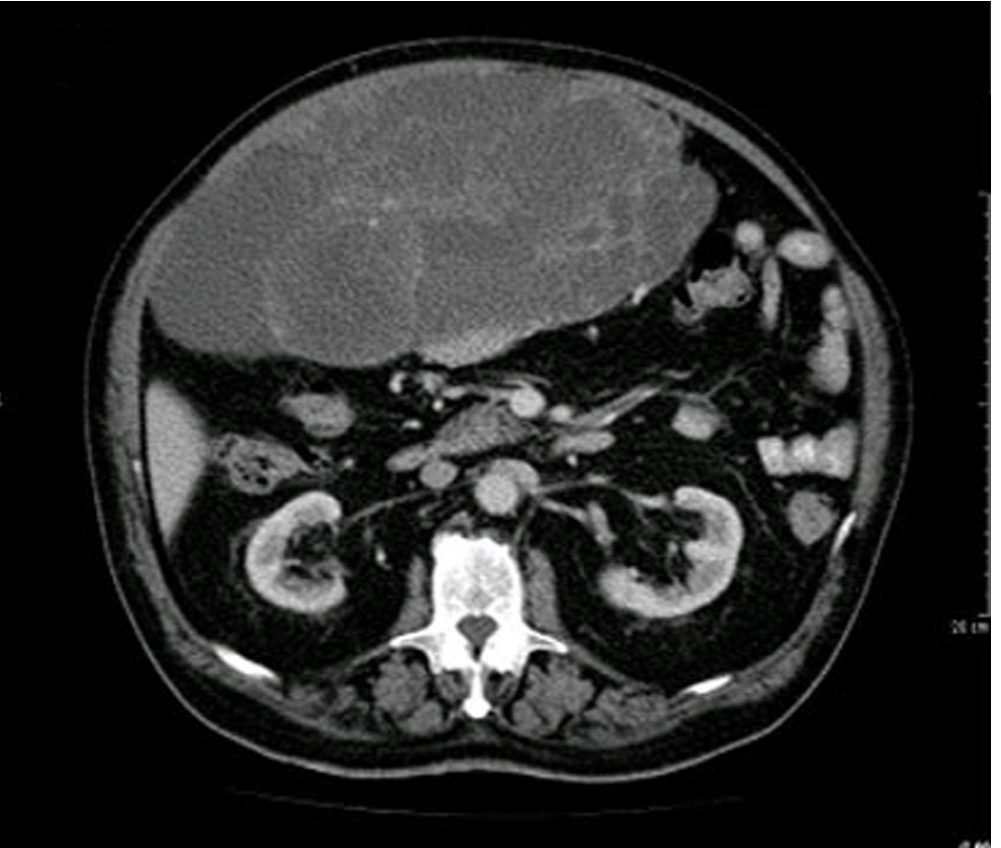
CT scan showing a voluminous intraperitoneal mass 33 × 30 × 17 cm in size, occupying the most part of the abdomen, with solid and cystic parts and with peripheral contrast enhancement.
